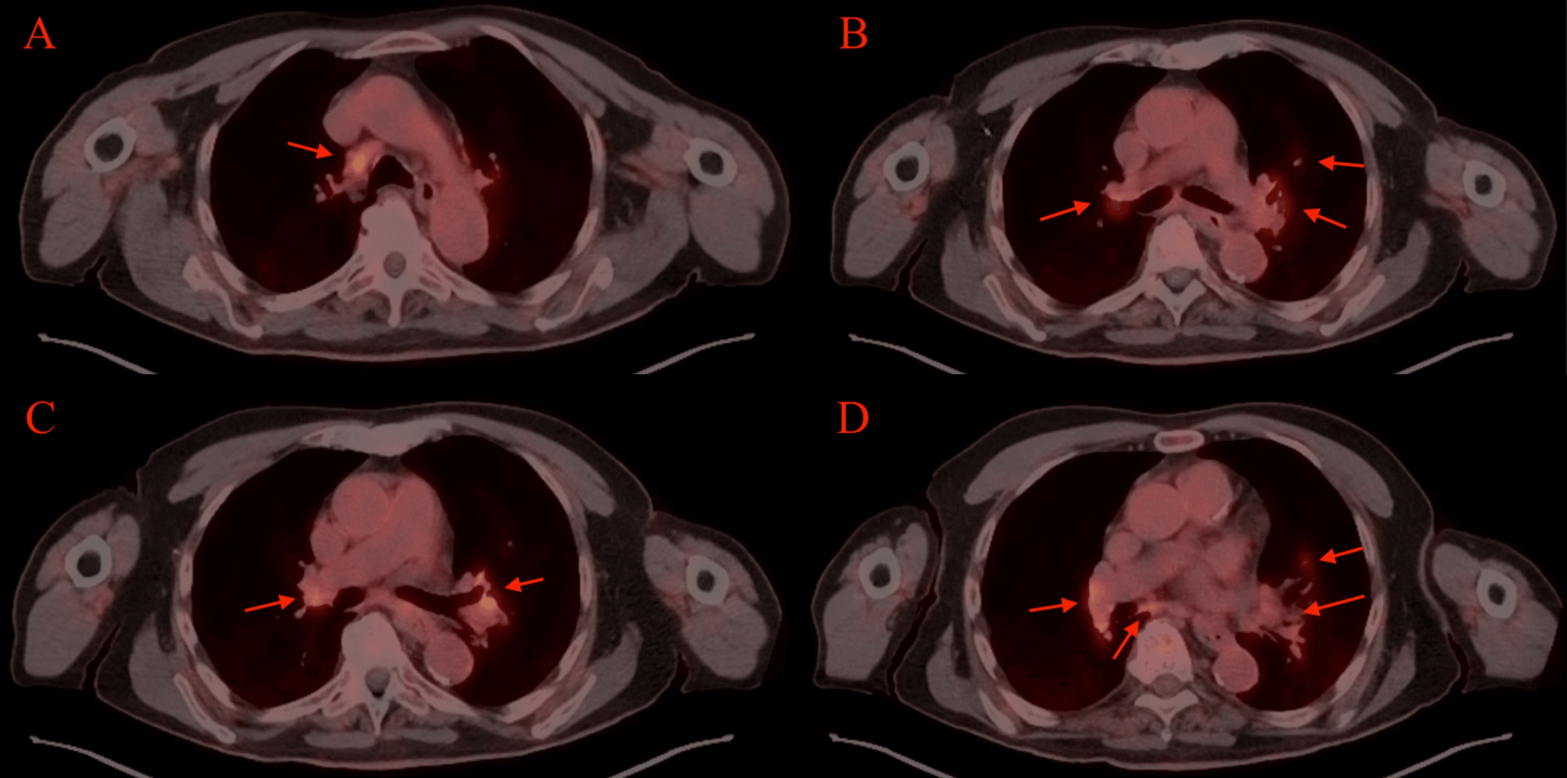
The PET scan demonstrated abnormal uptake in multiple bilateral pulmonary nodules (SUV 2.8), as well as in the superior mediastinal, pretracheal, subcarinal, and bilateral hilar lymph nodes (SUV 6.1). SUV: standardized uptake value, PET: positron emission tomography. SUV >2.5 often suggest malignancy. A-D represent sequential transverse PET scan sections from superior to inferior levels, respectively. A: Focal increased uptake in the right paratracheal or superior mediastinal lymph node region. B: Multiple bilateral increased uptake in the mediastinal, pretracheal, and hilar lymph node stations. C: Increased uptake is seen in bilateral hilar regions, consistent with lymphadenopathy or possible pulmonary nodules. D: Diffuse abnormal uptake persists in subcarinal and hilar nodes, with additional pulmonary involvement. Red arrows indicate areas of abnormal uptake.
